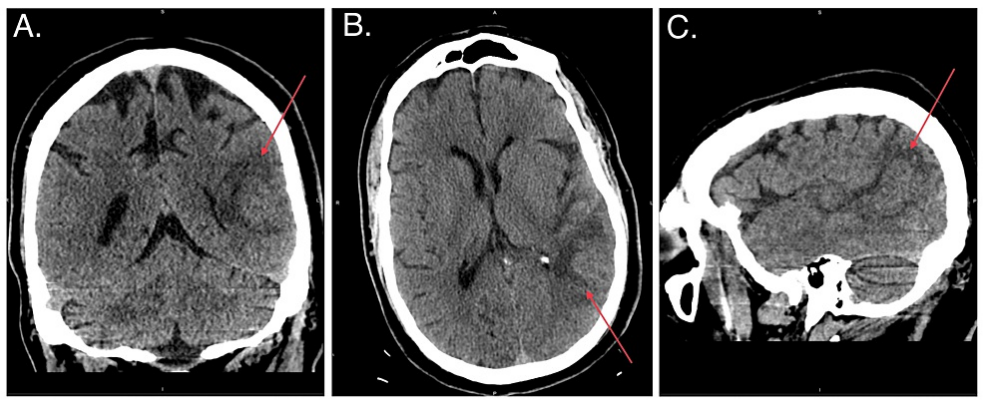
Head CT showing a 2.9-cm temporoparietal hypointensity with mild surrounding vasogenic edema (red arrows).
